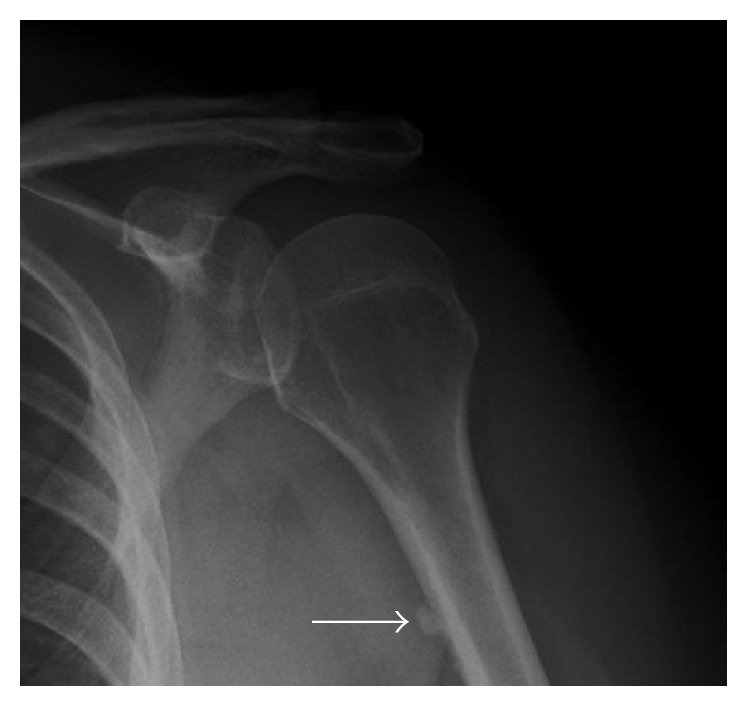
Calcific tendinopathy of the pectoralis major. Pectoralis major calcific tendinopathy can be seen along the anterior cortex of the proximal humerus diaphysis on lateral humerus or internal rotation AP shoulder radiographs (arrow).
